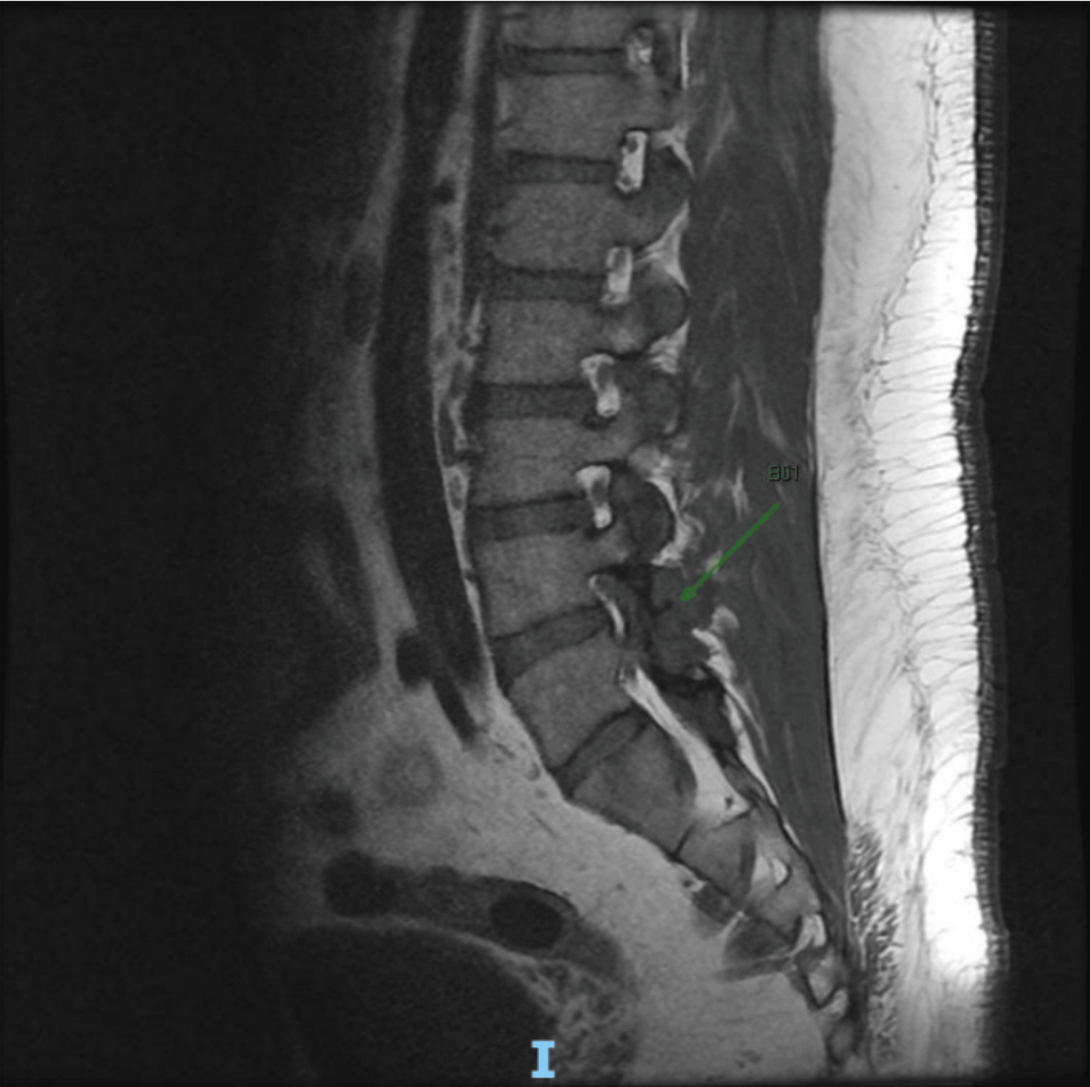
MRI Lumbar Spine Sagittal View, T1-Weighted
